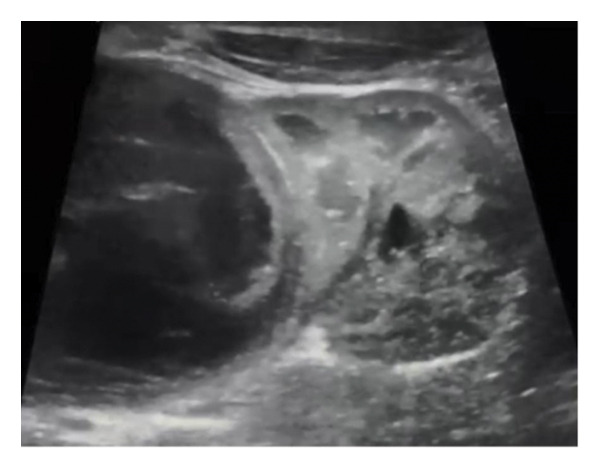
Follow‐up ultrasound (Day 36) showing enlargement and increased heterogeneity of the suprarenal cystic lesion.
